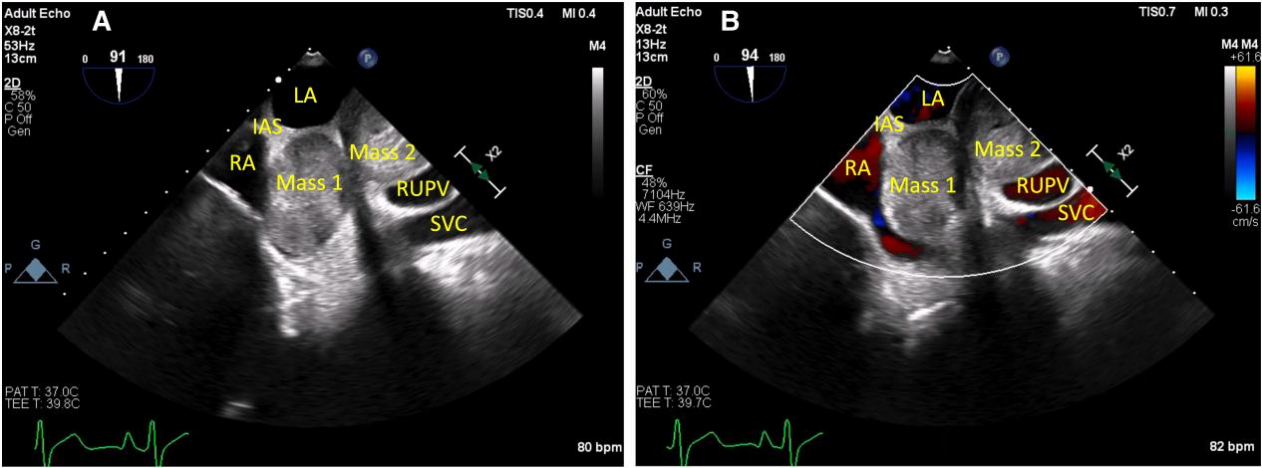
(*A*) Transoesophageal echocardiogram with (*B*) colour flow Doppler showing a large globular bilobed mass arising from the posterior aspect of the left atrium, (*A*) pushing onto the interatrial septum, (*B*) the posterior left atrium, and right pulmonary vein. IAS, interatrial septum; LA, left atrium; RA, right atrium; RUPV, right upper pulmonary vein; SVC, superior vena cava.
